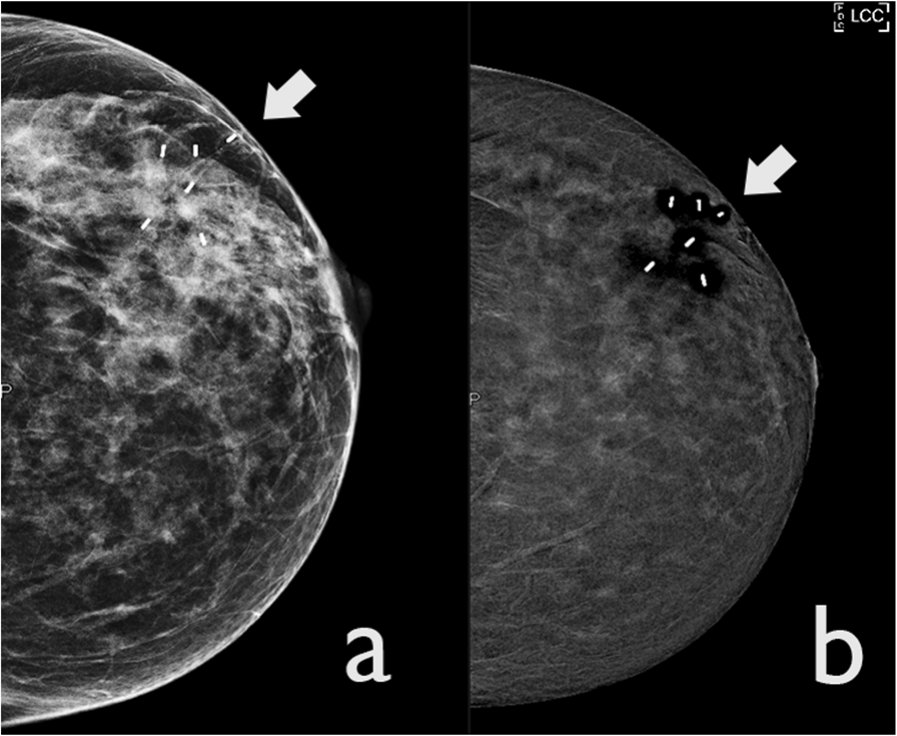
Artefact due to the presence of post ultrasound guided biopsy markers: a LE CEDM image. There are some markers seen at the outer quadrant of the left breast (arrow); b in the recombined image, the markers are seen as high attenuation structures and they demonstrate a surrounding dark halo
